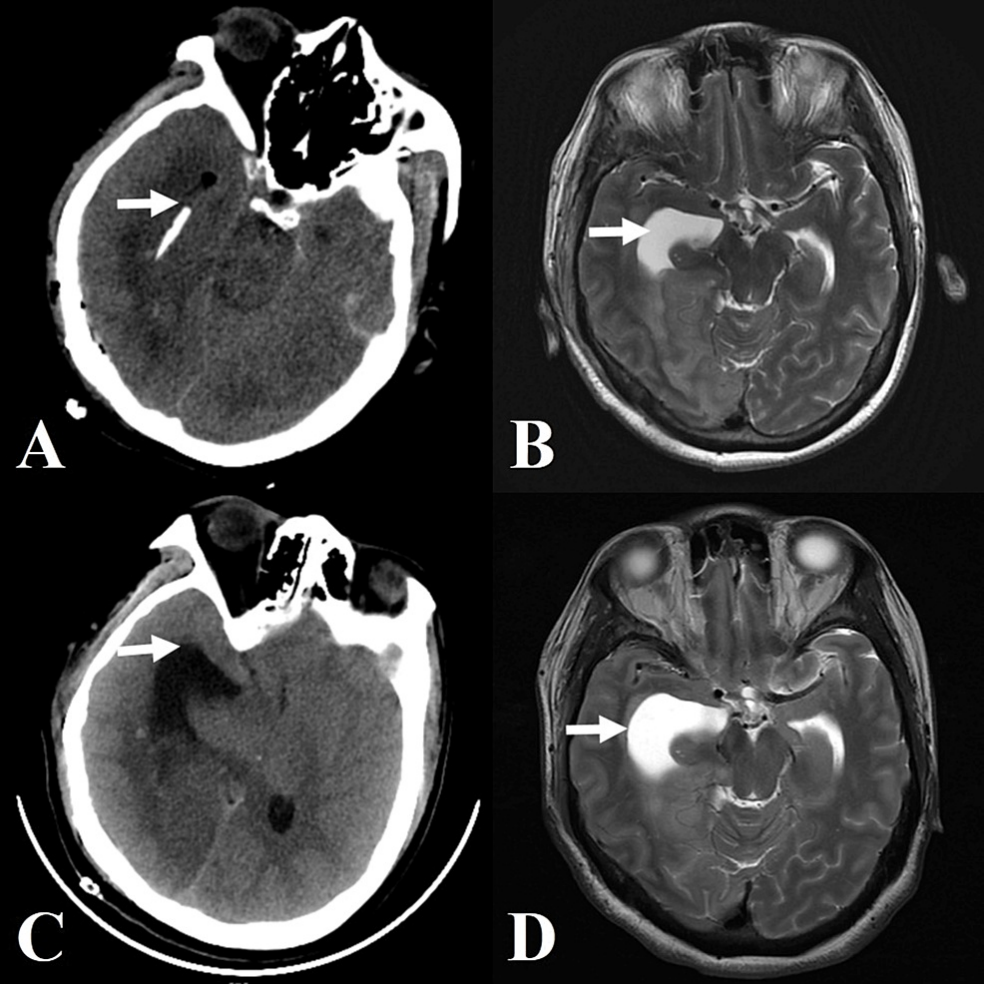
Image of gradual dilation of the temporal horn after surgery in Case 1 A: CT showed temporal horn stenosis on the first day after surgery, along with a narrowed left temporal horn, and the catheter was attached to the ventricle wall. B: MRI showed a right temporal horn width of 3.51 cm on the fourth day after surgery. C: CT scan showed a right temporal horn width of 3.80 cm on the eight day after surgery. D: MRI showed a right temporal horn width of 3.83 cm on the 11th day after surgery.
